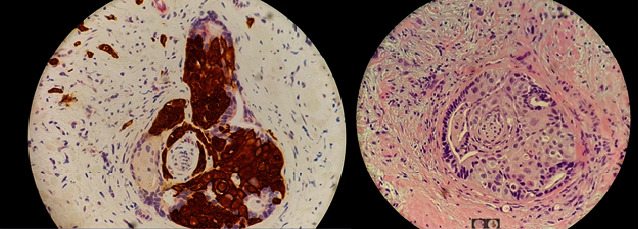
Axial chest CT scan showing a 5 mm solid pulmonary nodule suspicious for metastatic disease.
